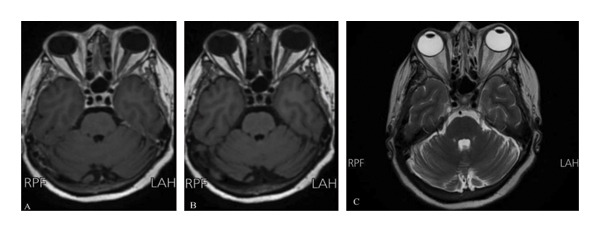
Brain MRI: T1‐weighted postcontrast (A), T1‐weighted precontrast (B), and T2‐weighted (C) images demonstrate no intracranial abnormalities or enhancing space‐occupying lesions.
